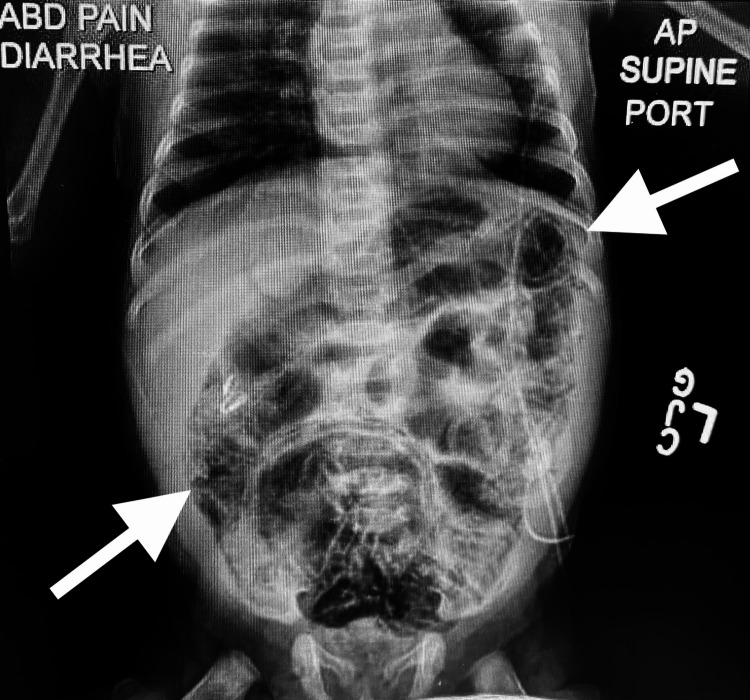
Abdominal x-ray demonstrates dilated loops of bowel and pneumatosis
